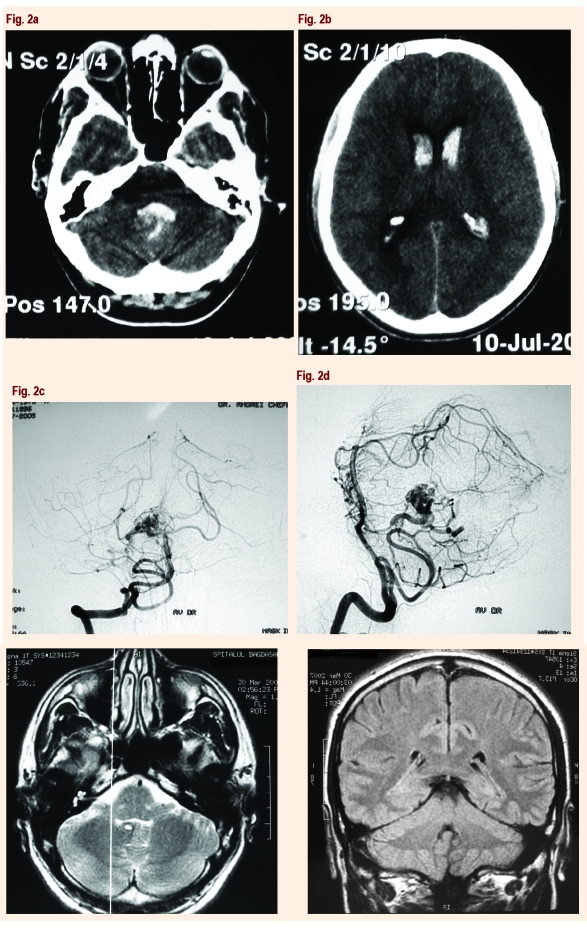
Pretreatment imaging studies of a 30–year–old man with basal panventricular hemorrhage as seen in axial CT in (a),(b); (c) anteroposterior and (d) lateral right vertebral angiogram showed a cerebellar AVM, located within the right cerebellar hemisphere, with a nidus, measuring around 1,5 cm maximal diameter, with arterial feeders from the right anteroinferior cerebral artery and venous drainage into both lateral sinus and straight sinus; axial (e) and coronal (f) MRI scan show a small vermian, right paramedian, arteriovenous malformation  with minimal mass effect.
